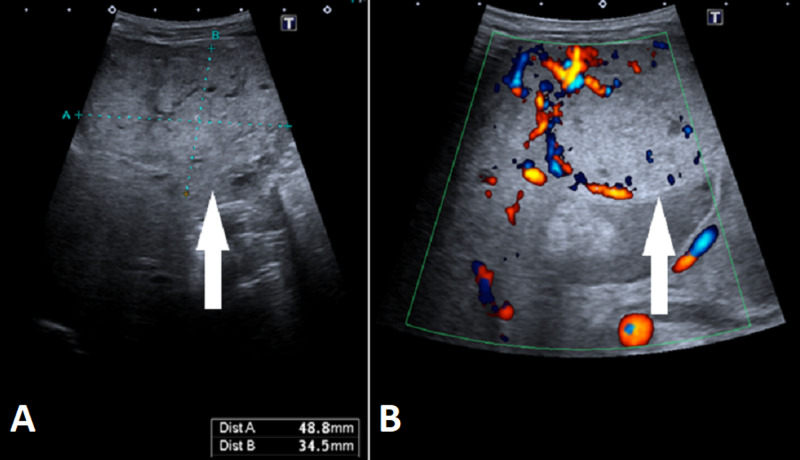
Abdominal ultrasound (US) (A) An abdominal US showing the size of the lesion (white arrow, blue dotted lines – distance A and B). (B) An abdominal Doppler US showing a hypervascular lesion (white arrow) with predominant peripheral vascularization.
